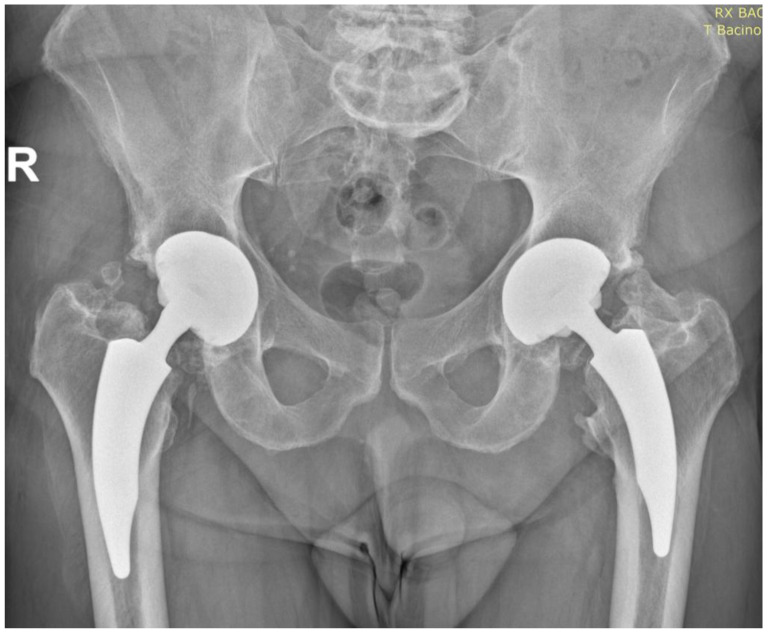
Postoperative AP radiograph at 15 years demonstrating stable fixation of the Nanos^®^ stem and R3™ cup, with heterotopic ossifications.
